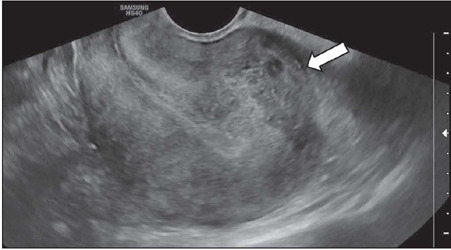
Transvaginal ultrasound image showing a retroverted uterus with adenomyosis
